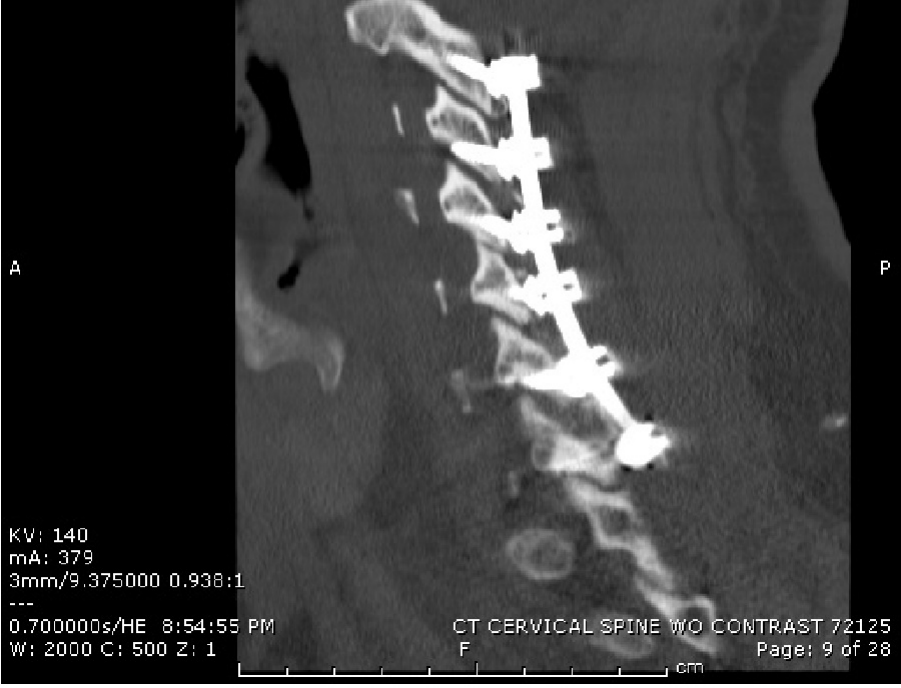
Postoperative sagittal cervical spine CT Intrumentation from C2-C7
